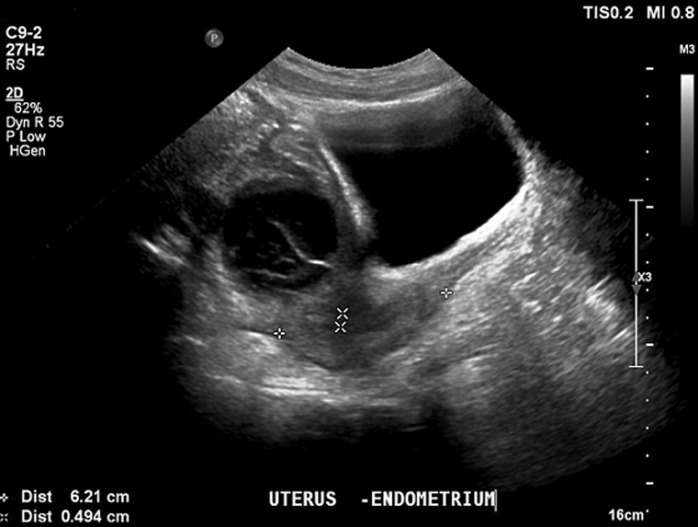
hemorrhagic ovarian cyst features on ultrasonography; ultrasonography revealing an ovoid cystic mass, with regular borders (47 x 45 x 56 mm), hyperechoic elements (possibly hemorrhagic features), multiple internal septations and absence of peripheral or internal vascularity
